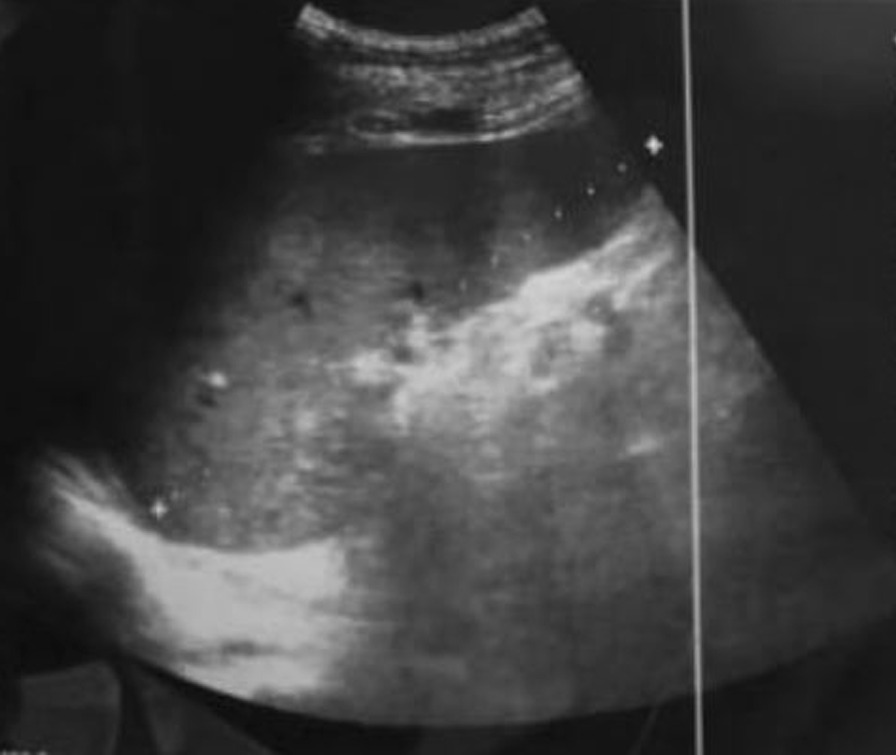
Pelvic ultrasound demonstrating an enlarged uterus with a heterogeneous mass obliterating the endometrial cavity, with a vesicular appearance
